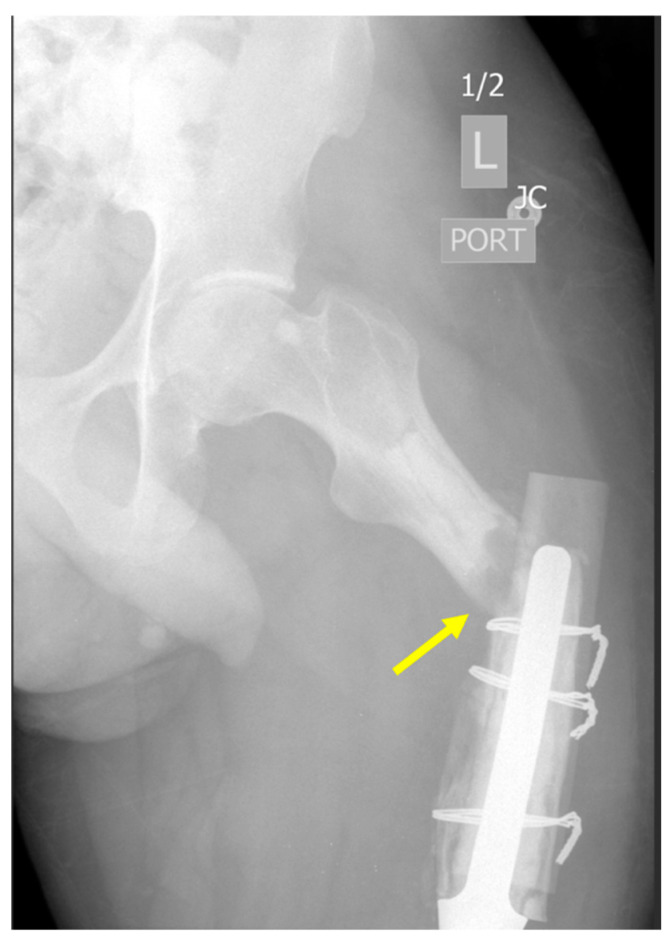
Plain radiograph of a patient with history of osteosarcoma treated with resection and endoprosthetic reconstruction, now presenting with frank periprosthetic fracture, UCS Type B2 (yellow arrow).
